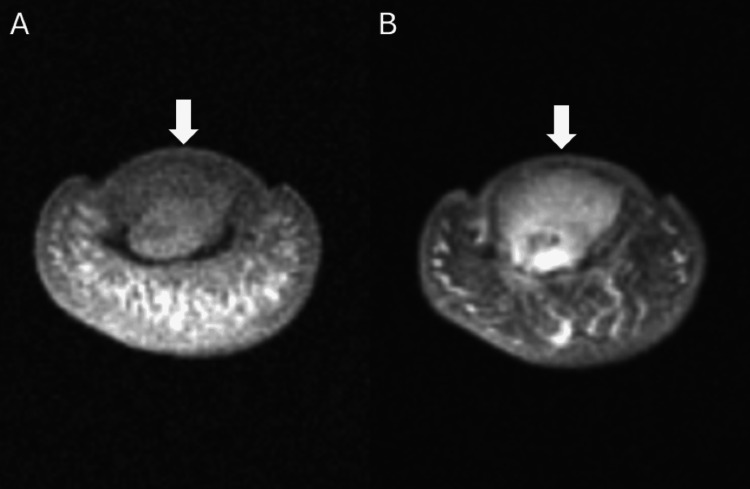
A) Axial T1 fat sat MRI of the left third distal phalanx demonstrating a solid lesion. B) Axial T1 fat sat post-contrast MRI of the left third distal phalanx of the distal phalanx, demonstrating a solid enhancing lesion.
